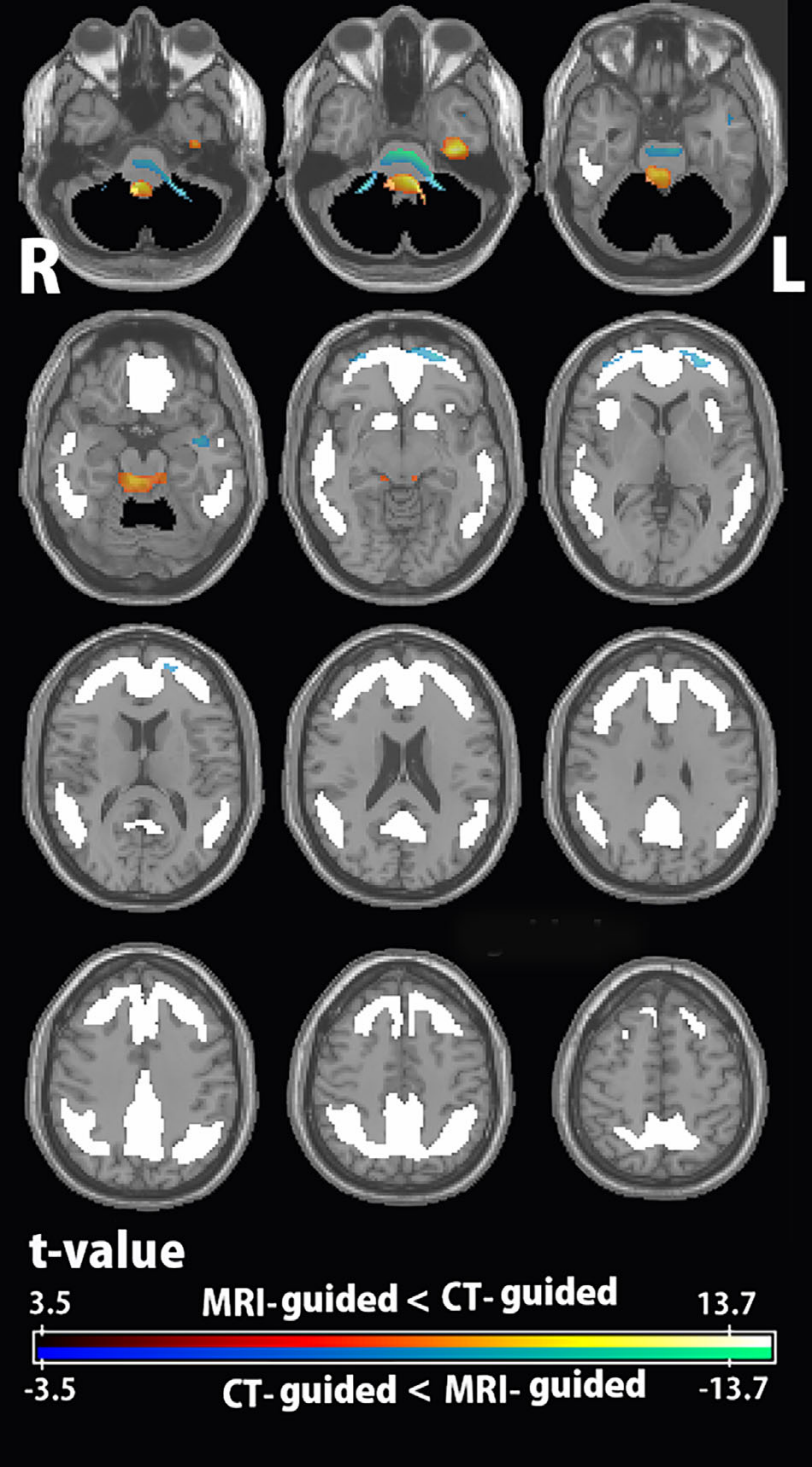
Direct comparison of anatomically standardized amyloid PET images using low‐dose CT and MRI statistical parametric mapping (SPM) analysis showed significantly (family‐wise error, *p* < .05) higher and lower uptake of CT‐guided standardized PET images than MRI‐guided standardized PET images, presented in a warm color scale and a cool color scale, respectively. Volume of interest (VOI) templates are shown as a solid black area for the whole cerebellum as a reference area and as a solid white area for the target cortical area. The largest differences in accumulation are visible in the brain stem. Lower uptake of CT‐guided standardized PET images was observed in the frontal cortex of the target cortical VOI.
